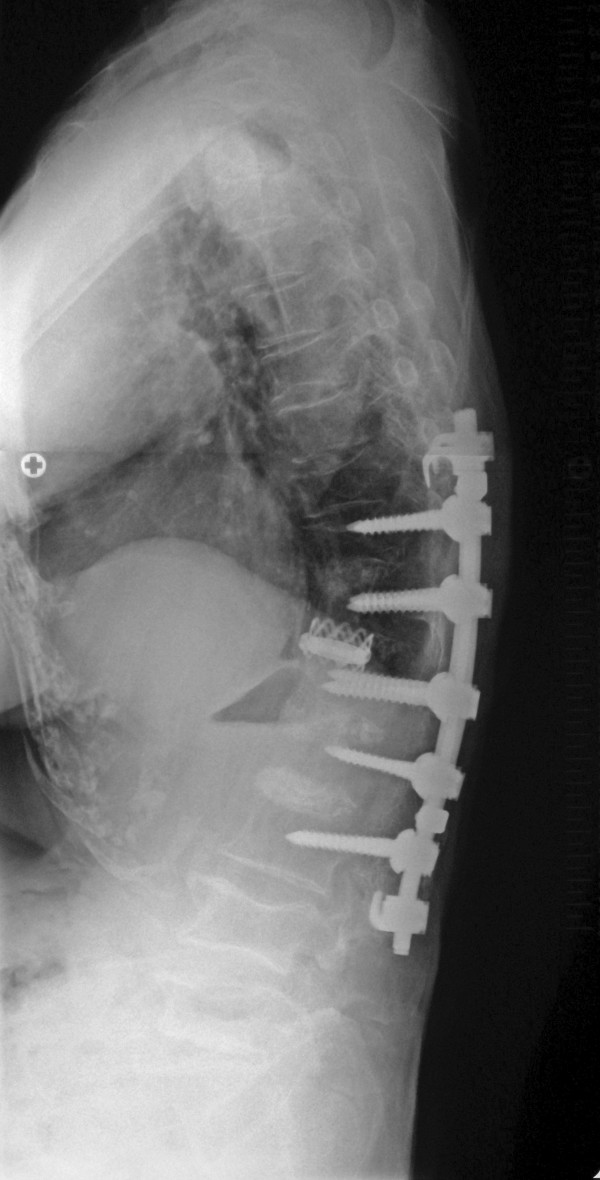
Standing lateral radiography of the spine obtained 12 years after surgery. No correction loss was observed up to final follow-up.
